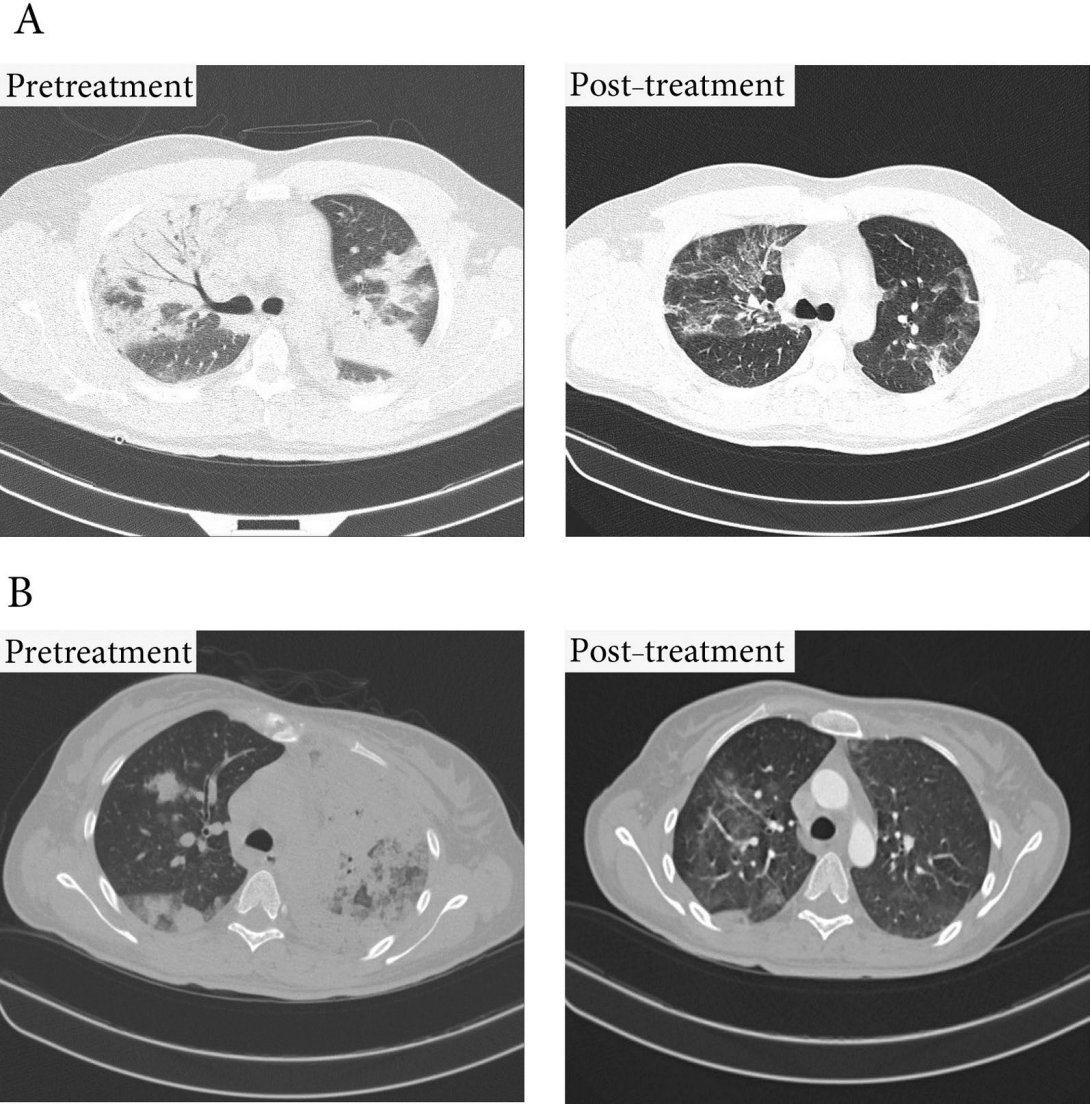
Lung CT scan. A Significant improvement in lung lesions within 10 days of starting treatment in a 37-year-old male patient B Significant improvement in lung lesions within 5 days of starting treatment in a 45-year-old female patient
